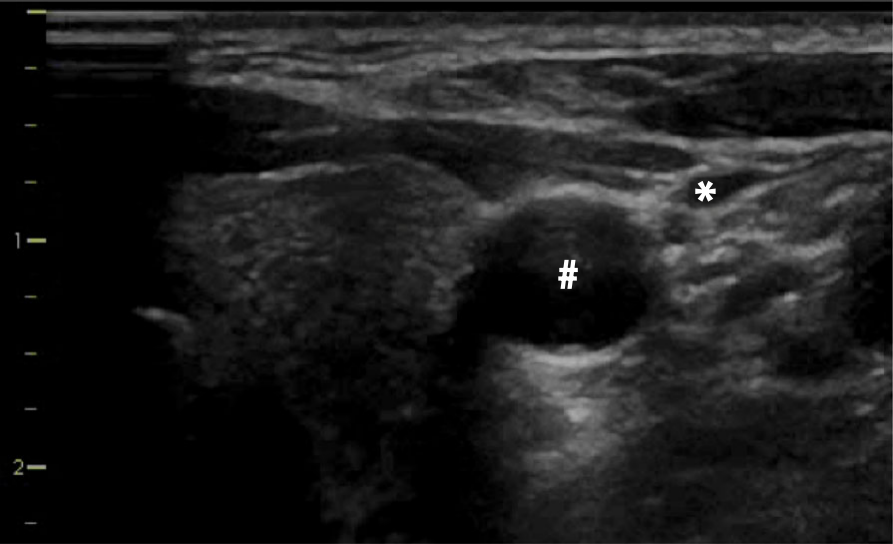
Ultrasound view of a small internal jugular vein. Short-axis (transverse) view of a small right internal jugular vein (*) and its anatomic relation to the carotid artery (#) (e.g., in a patient with intravascular hypovolemia)
